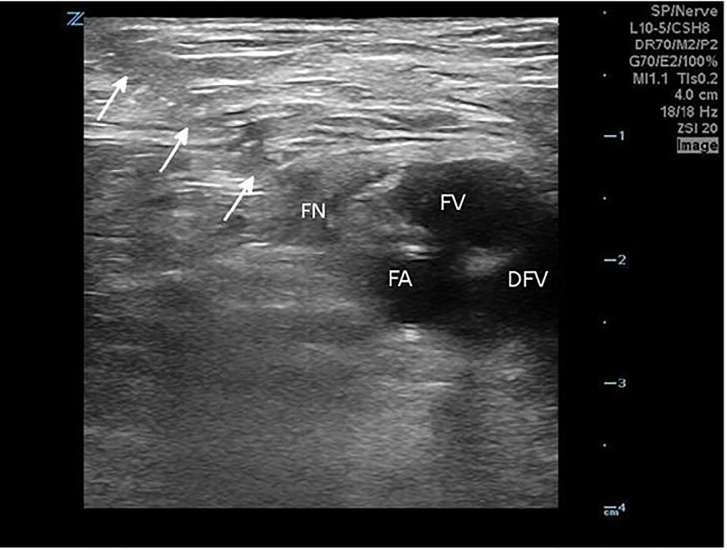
Ultrasonographic image of the right femoral nerve and associated vascular structures, obtained during injection of anesthesia around the femoral nerve. The series of arrows outlines the course of the needle inserted for delivery of the anesthetic. *FN*, femoral nerve; *FA*, femoral artery; *FV*, femoral vein; *DFV*, deep femoral vein.
